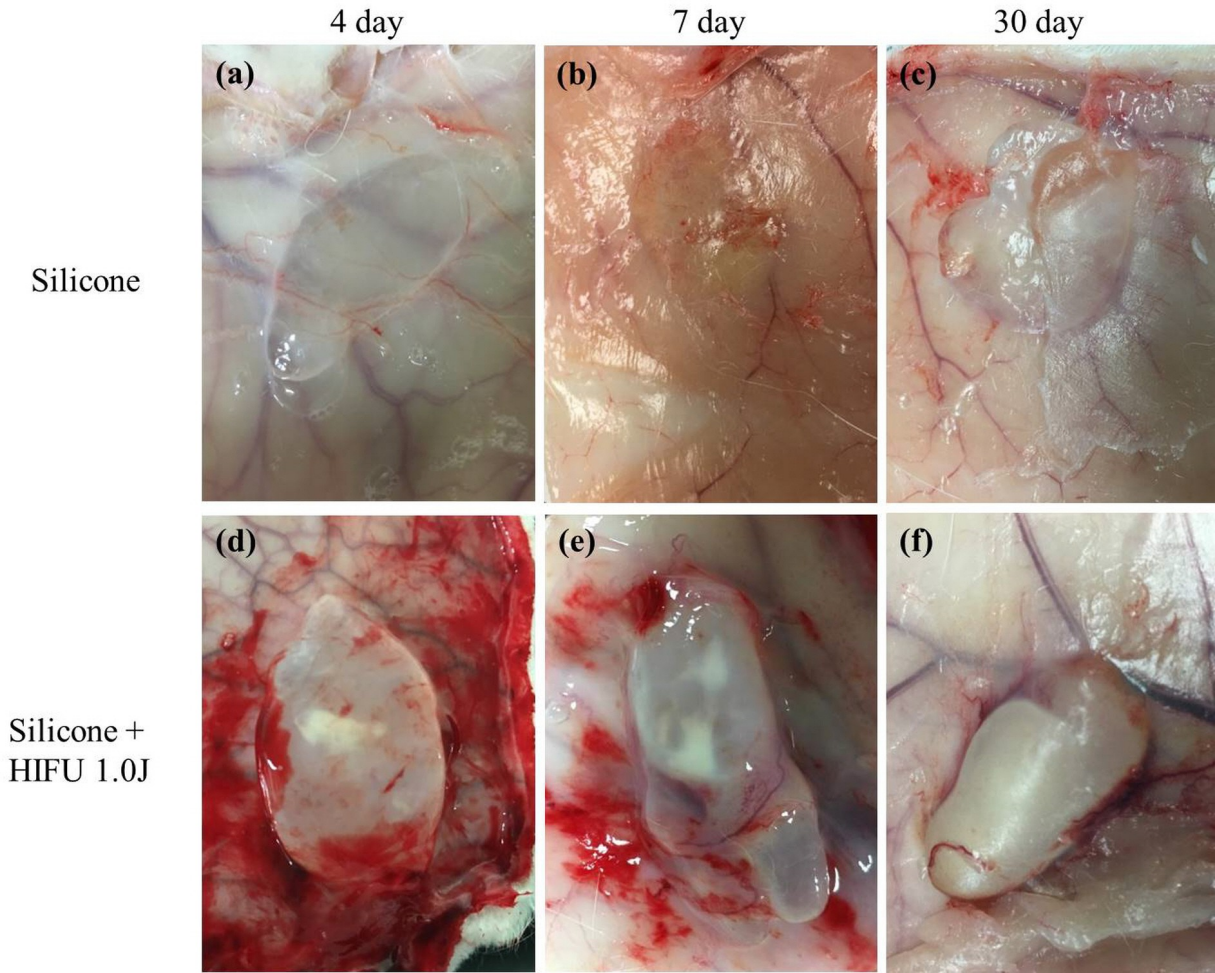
Gross results of high-intensity focused ultrasound (HIFU) treatment after silicone injection. Images collected on Days 4, 7, and 30 after HIFU treatment, to compare tissues from the normal control group (untreated) and the experimental group (treated with HIFU). After each rabbit was euthanized, we evaluated the inner side of the skin (a, b, c). In the control group, the injected silicone was flattened without signs of inflammation, and the capsules were not clearly visible to the naked eye. There was no noticeable difference between the tissues from Days 4, 7, and 30. (d) Visual examination of the tissue from the experimental group on Day 4 after HIFU treatment. Compared with the control group, clear formation of a capsule occurred. (e) Visual examination of the tissue on Day 7. The capsule was more obvious and opaque. (f) Visual examination of the tissue on Day 30. The thickness of the capsule had increased, and progressive fibrosis was obvious. Vascular structure was visible on the outer layer of the capsule. Compared with the normal control group, in which the capsule was flattened, the capsule in the experimental group was bulky and protruding.
